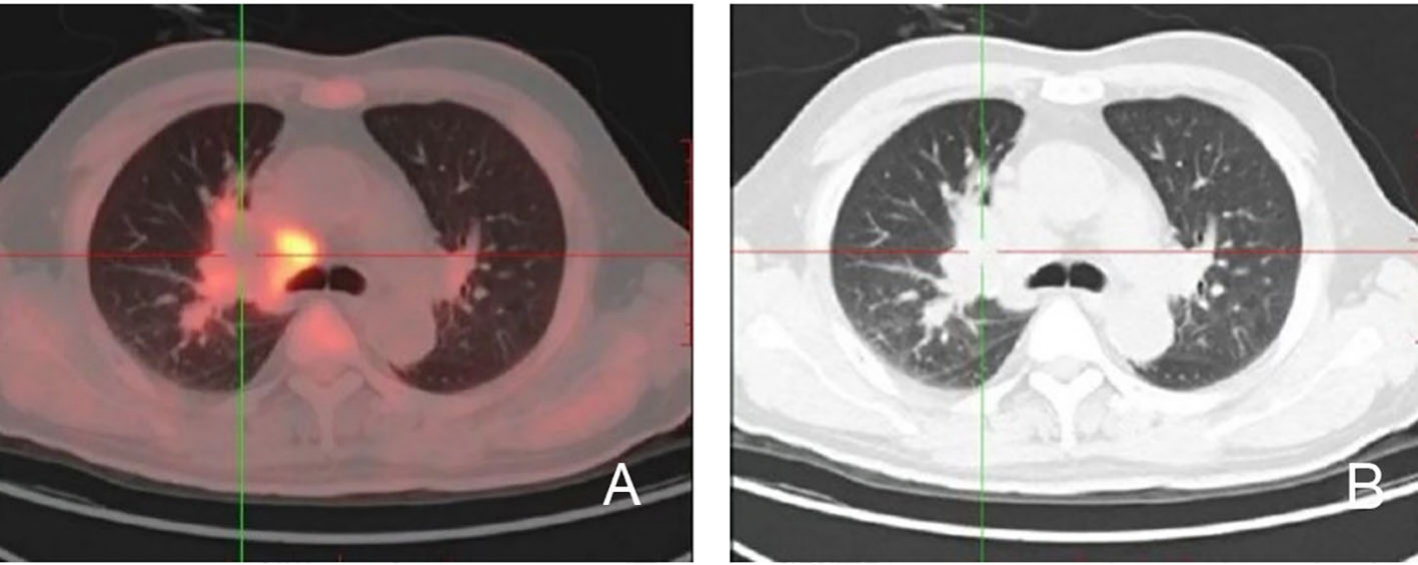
PET-CT imaging of primary lung extranodal NK/T-cell lymphoma. (A) The CT component of the PET-CT scan shows an irregular mass in the right hilum, adjacent to the pulmonary vessels, with evidence of local invasion and airway compression. The lesion is characterized by irregular borders and lobulated appearance. (B) The fused PET-CT image reveals intense FDG uptake in the same right hilar mass, indicating hypermetabolic activity, consistent with a malignant process. The high SUV suggests aggressive tumor behavior. Increased FDG uptake is also visible in the surrounding tissue, raising concerns for possible lymphatic or adjacent structure involvement. CT, computed tomography; ENKTL, extranodal NK/T-cell lymphoma; FDG, fluorodeoxyglucose; PET-CT, positron emission tomography; NK/T-cell lymphoma; NK, natural killer; SUV, standardized uptake value.
